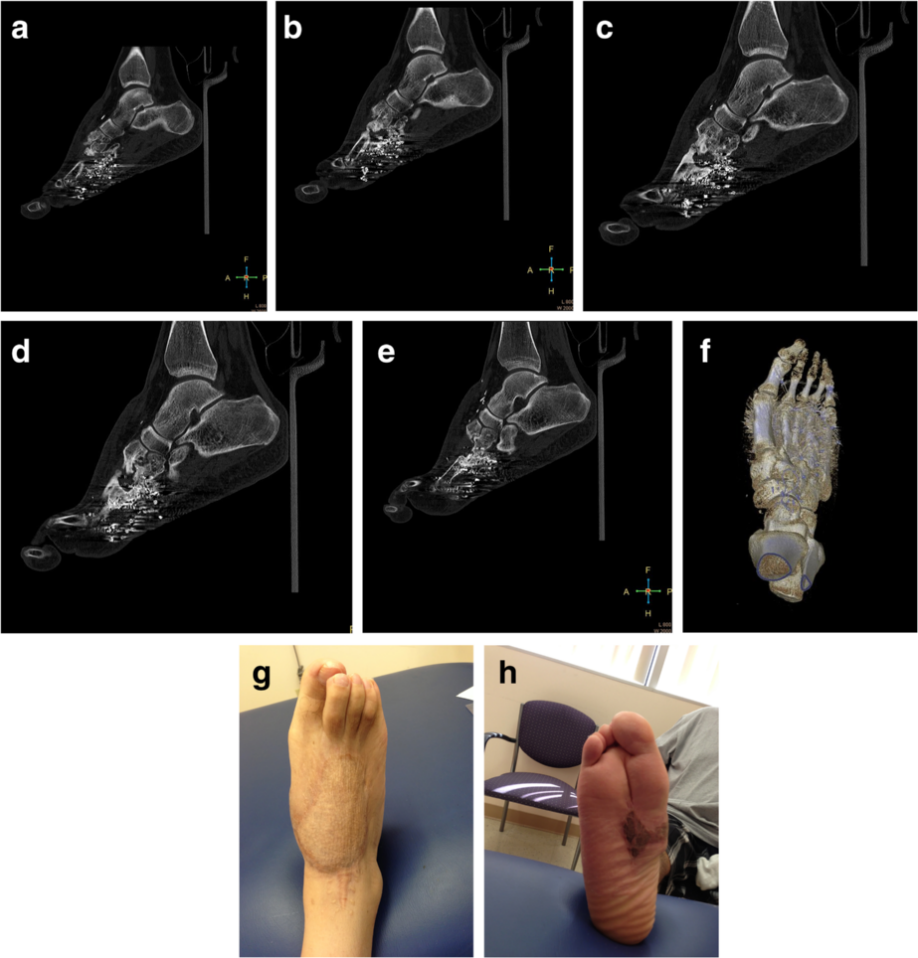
Computed tomographic (CT) and photographs of the right foot. Sagittal (a-e) and CT reconstructions (f) of the right foot approximately 3 years post-op. Photographs (g-h) of the right foot 3 years post-op.
